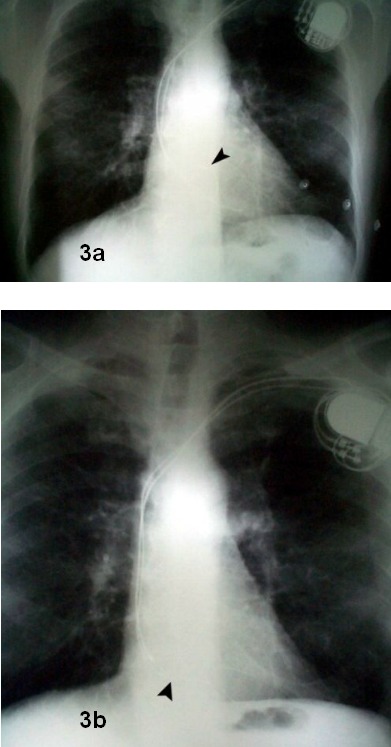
Posteroanterior chest x-ray film. Top (A): radiograph obtained 24 hours after pacemaker implantation. Arrowhead shows atrial lead tip inside the right atrial appendage. Bottom (B): radiograph obtained three months later, showing displacement of atrial lead towards tricuspid annulus. However, diagnosis is not evident from the posteroanterior view alone, due to superimposition of structures and radiograph densities.
